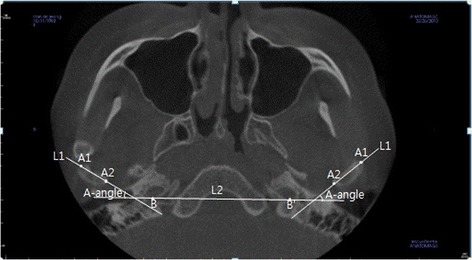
Reference points and measurement of axial view in CBCT. A1 outer pole of condylar head, A2 inner pole of condylar head, B,B′ hindmost point of the carotid canal, L1 line passing A1 and A2, L2 line passing B and B′, A-angle angle between L1 and L2
